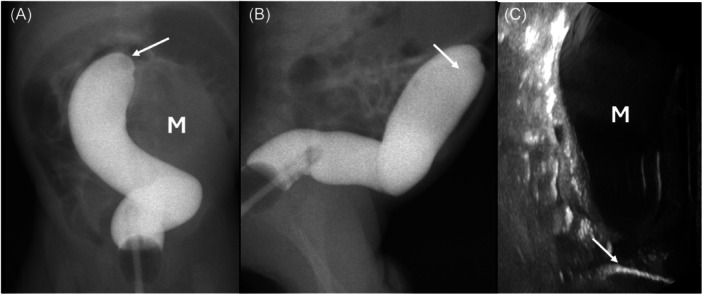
A high‐pressure contrast enema on anteroposterior (A) and lateral view (B) exhibited a blind‐ended sigmoid colon (arrow) with a mass‐like soft tissue density at left‐sided sigmoid colon (M in A). Additional ultrasound (C) revealed a fluid‐filled bowel loop proximal to the blinded end (arrow) of the sigmoid colon (M in C), suggestive of sigmoid colonic obstruction.
